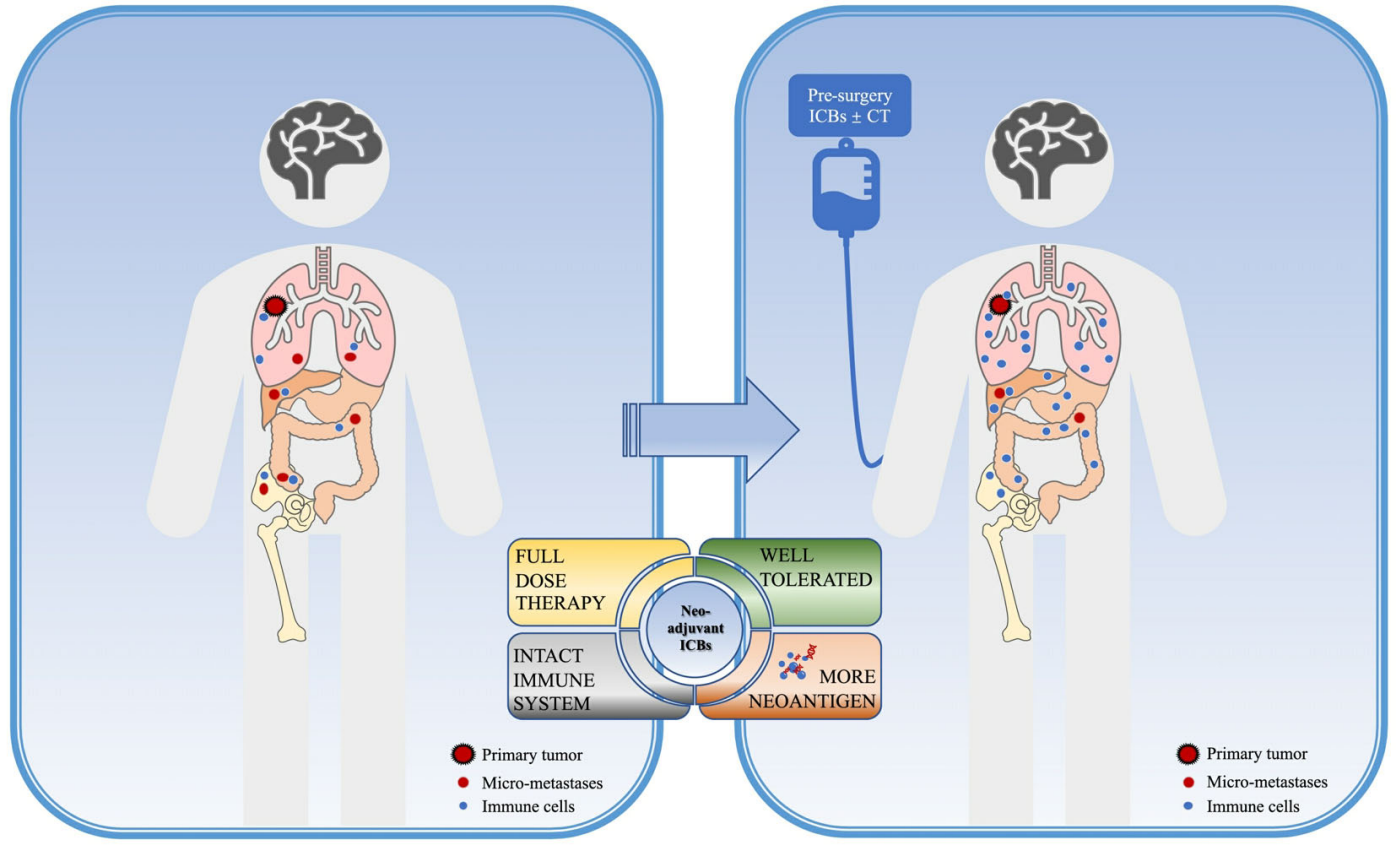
Rationale of neoadjuvant immunotherapy. Neoadjuvant immune checkpoint inhibitors (ICBs) in NSCLC might provide higher benefits for non-small cell lung cancer (NSCLC) patients due to the following points: before surgery, the primary tumor and distant micro-metastases providing a pleural of tumor-specific neoantigens, with the intact immune system, the immune response could be of the greatest work, which could obliterate micro-metastases in return. Also, better performance status before surgery could offer higher opportunities for patients to receive a full-dose systemic therapy with well tolerance. ICB, immune checkpoint inhibitors; CT, chemotherapy.
